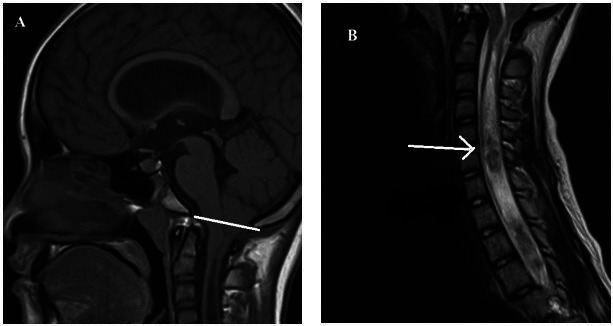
(A) Sagittal magnetic resonance imaging (MRI) showing Chiari malformation 1 (CM 1). The white line represents the McRae line connecting the basion and opisthion. (B) T2-weighted sagittal MRI displaying a cervical-thoracic syrinx, indicated by the white arrow.
